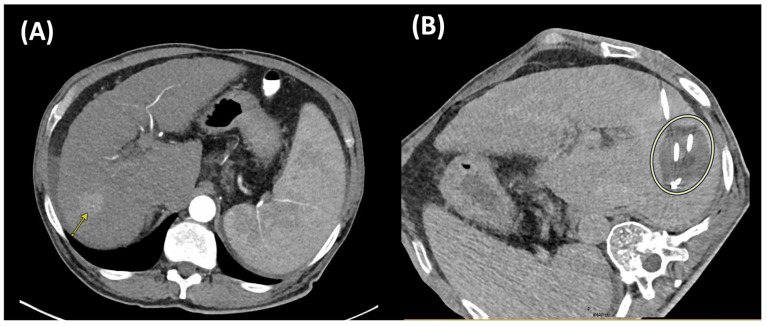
Axial CT imaging before and during cryoablation. (A) Pre-treatment scan demonstrating a 2.7 cm arterially enhancing HCC in the right lobe (arrow). (B) Intraprocedural CT during cryoablation with four applicators, obtained during the second freeze cycle, showing the characteristic ice ball measuring 4.5 × 4.0 cm (yellow circle).
